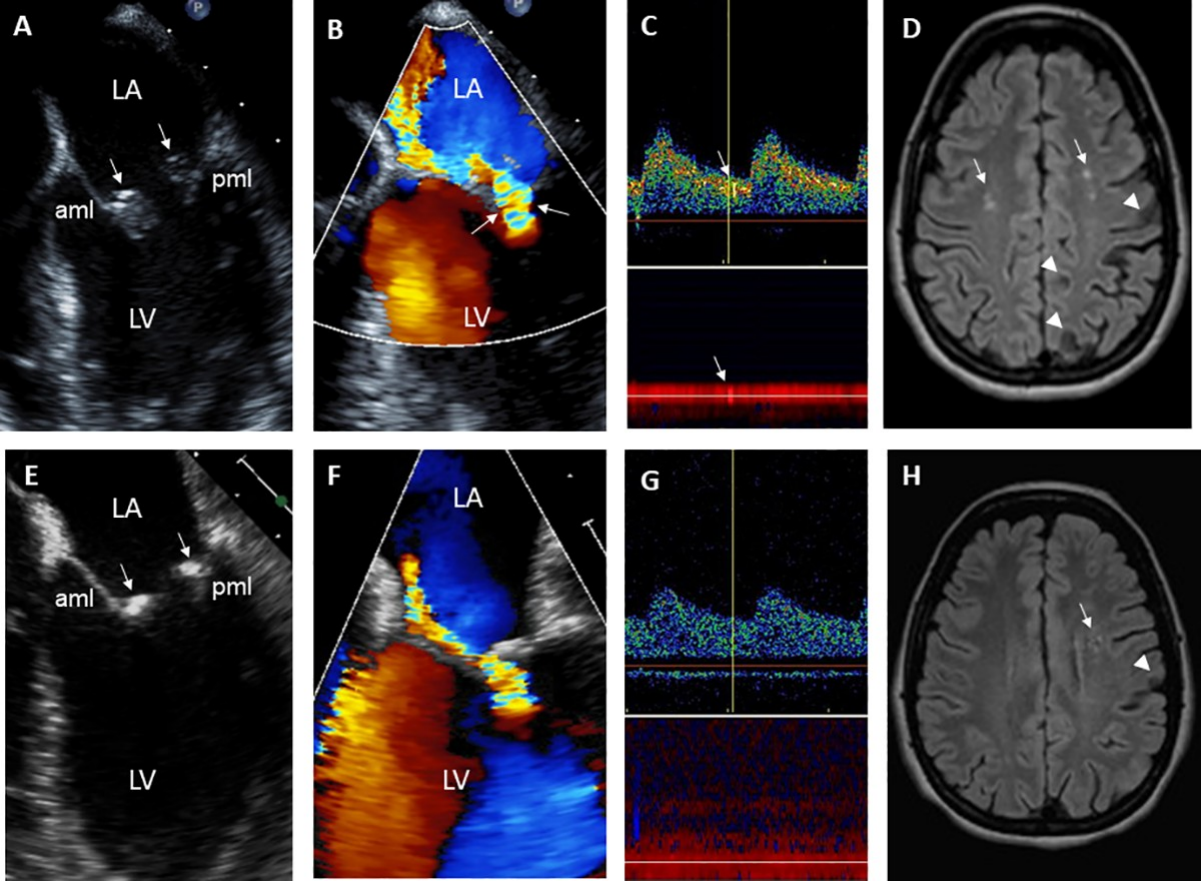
50 year old female with Libman-Sacks endocarditis complicated with an acute transient ischemic attack, past stroke, and seizure disorder. A,B. These 2D and color-Doppler TEE views demonstrate moderate size (area of 0.85 cm^2^), sessile, and oval shaped Libman-Sacks vegetations (arrows) on the distal portions and atrial side of the anterior (aml) and posterior (pml) mitral leaflets (A) associated with moderate to severe highly eccentric mitral regurgitation (B). C. Transcranial Doppler of the middle cerebral artery demonstrates 1 of 2 microemboli (arrows). D. MRI of the brain demonstrates 3 cerebral infarcts (arrowheads) and multiple small periventricular and deep white matter abnormalities (arrows) for a lesion load of 1.2 cm^3^. Her global neurocognitive dysfunction was graded as moderate. E,F. After 5 months of warfarin, aspirin, hydroxychloroquine, and steroids, repeat TEE demonstrated significantly smaller (area 0.13 cm^2^) and homogeneously hyperreflectant indicative of healed mitral valve vegetations (E) and improved mitral regurgitation to moderate in degree (F). G,H. She also had no cerebromicroemboli (G), her number of brain lesions (H) and lesion load decreased to 0.7 cm^3^, and her neurocognitive dysfunction improved to mild degree.
